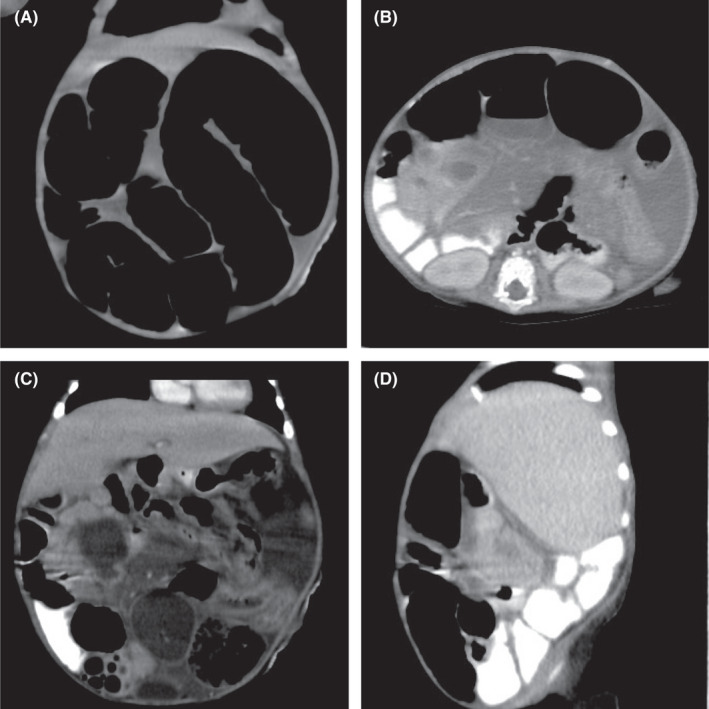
(A) CT; Dilated Bowel loops. (B) CT; thickened bowel wall and mesentery. (C) CT; thickened bowel wall and mesentery. (D) CT; thickened bowel wall and mesentery
